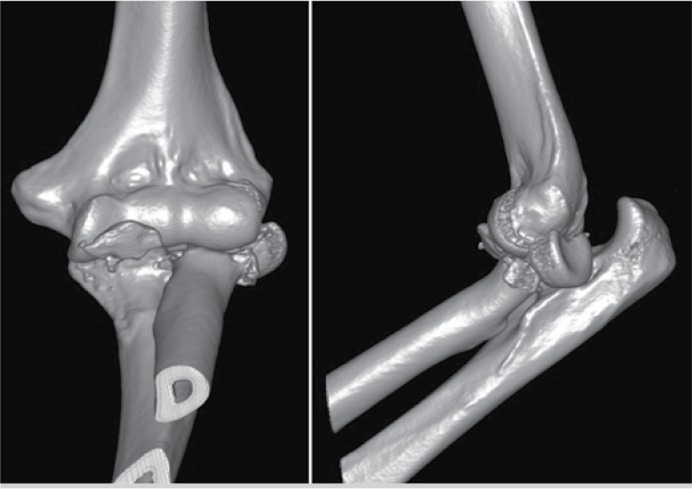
Computed Tomography (3D reconstruction) of the injury.
